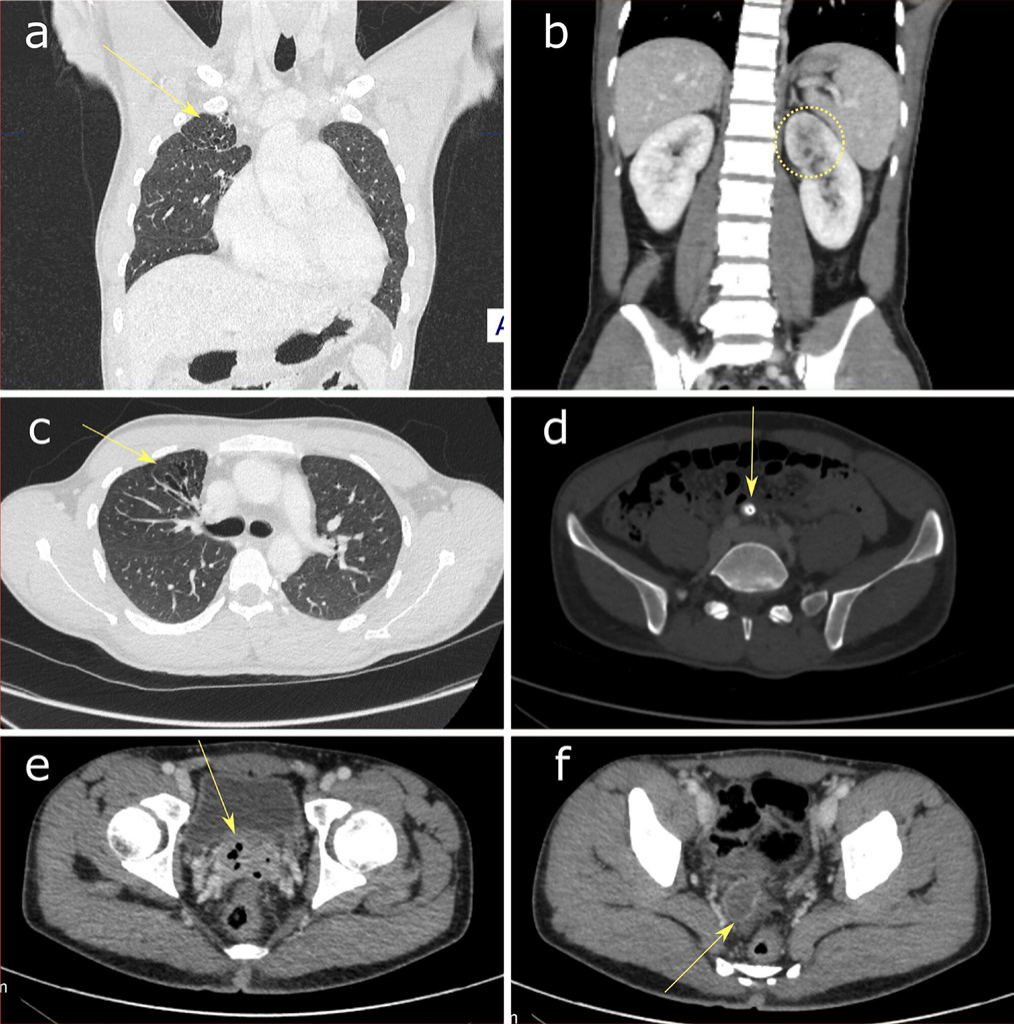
Contrast-enhanced CT Imaging. (a, c) Coronal and axial sections of the thorax in lung windows. There is right apical reticular change with pleuroparenchymal distortion and associated bronchiectasis (arrows). (b) Coronal view through the kidneys demonstrates an ill-defined left upper pole low attenuation hypoenhancement (dotted region), with normal enhancement of the remainder of the renal parenchyma bilaterally. (d) Axial view through the pelvis demonstrates a solitary peripherally calcified retroperitoneal lymph node (arrow). (e) Axial view through the deep pelvis demonstrates multiple small foci of gas within the prostate gland (arrow). (f) Axial view in the mid-pelvis demonstrates a peripherally enhancing low density collection in the region of the right seminal vesicles.
